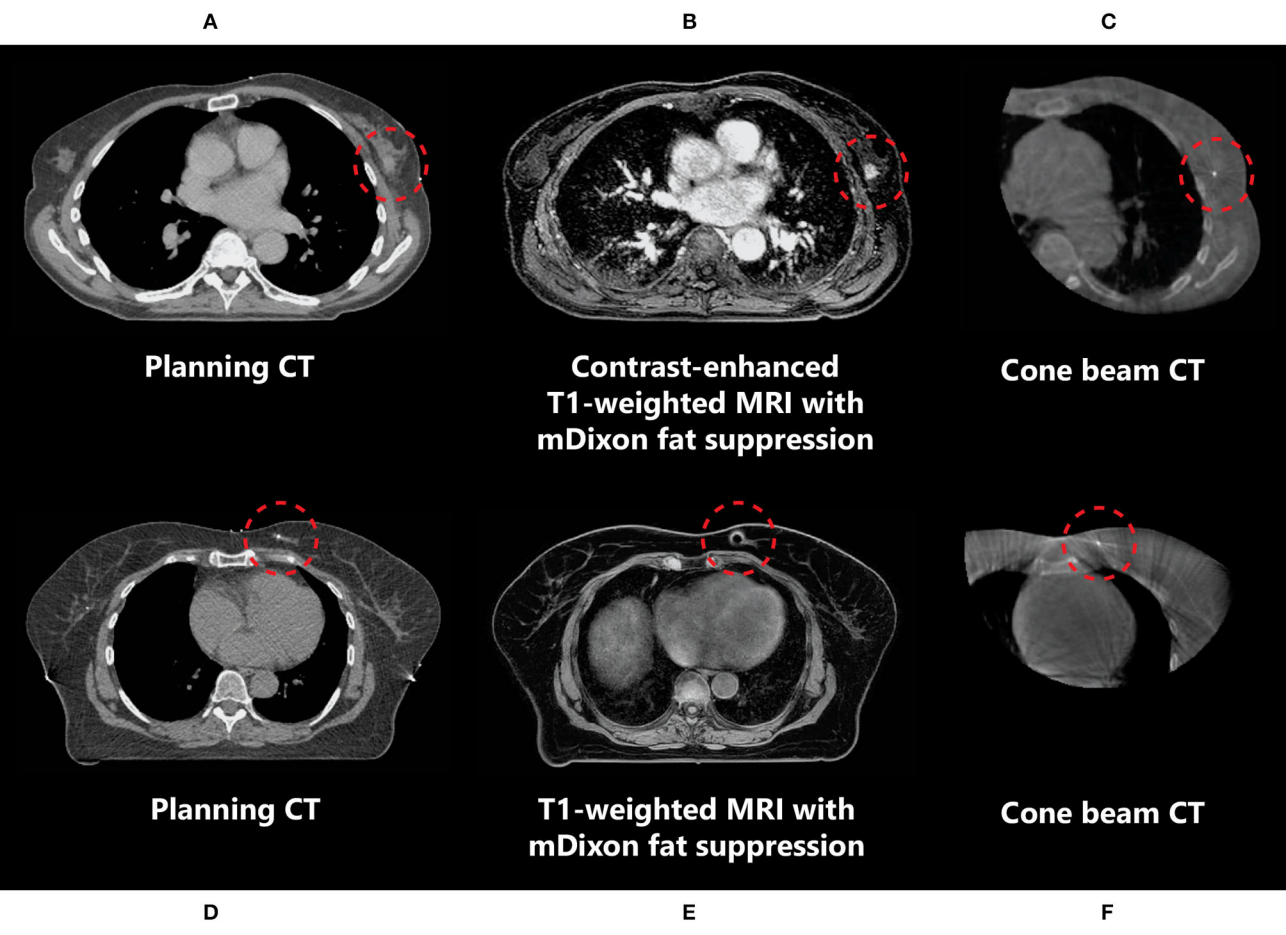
Imaging of a primary breast tumour on CT (A,D), (contrast-enhanced) MRI (B,E), and CBCT (C,F) scans indicating the difference in tumour visibility (inside the red circle) between these modalities in two different patients (A–C and D–F). (D–F) The marker inserted in the tumour medial in the left breast is observed as a void on MRI (indicated by the red circles).
